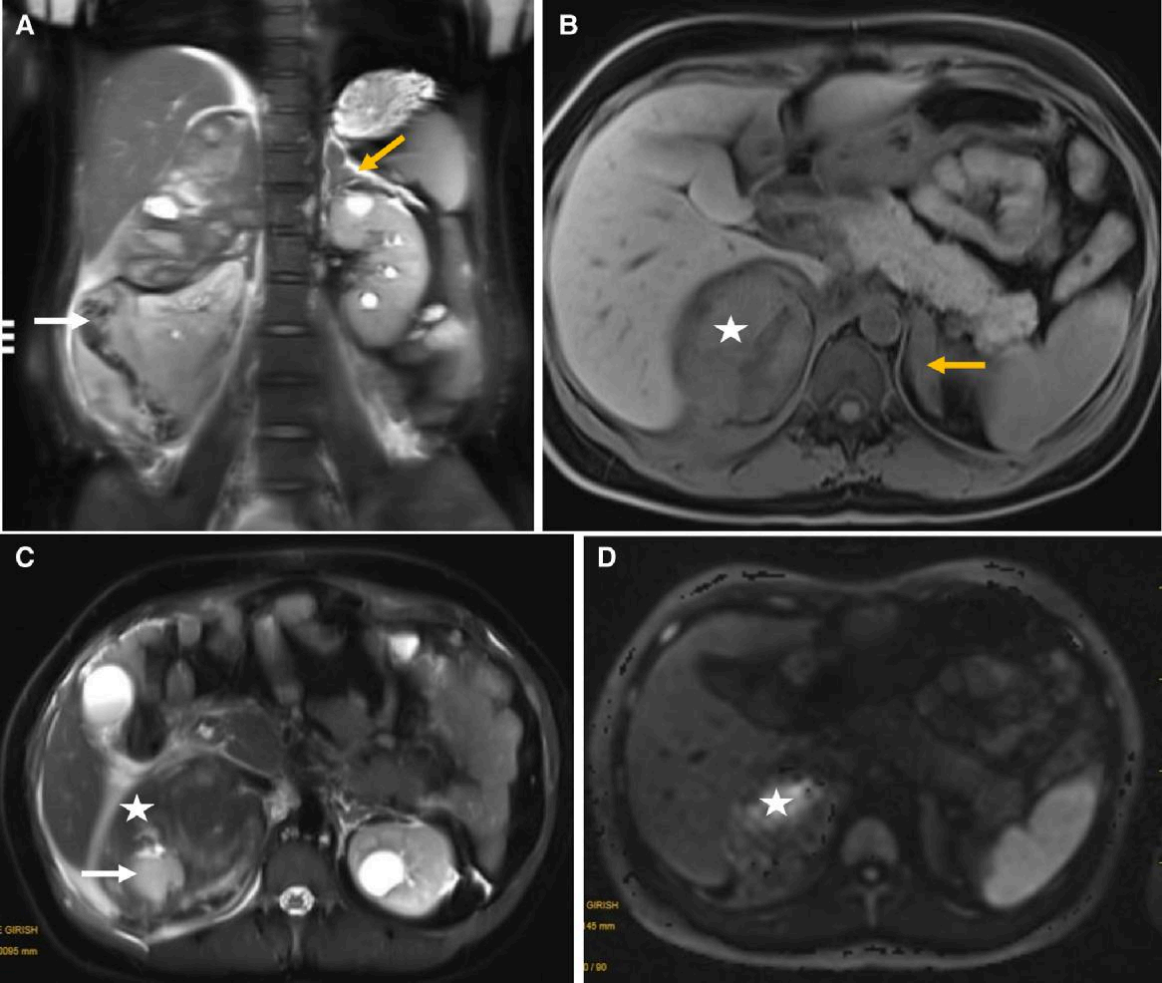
Magnetic resonance imaging showing a well-defined lesion (8.7 × 8.2 × 11 cm) in the right suprarenal area that was heterogeneously hyper-isointense on T2-weighted half Fourier single-shot turbo spin-echo coronal image (A), hyperintense with no fat suppression on axial T1-weighted fat saturation sequences (B) with breach in the posterior capsule with fluid/clots in the right posterior perinephric and pararenal space suggestive of right adrenal hemorrhage (white arrows) (A,C). Corresponding patchy areas of diffusion restriction representing acute hemorrhage (white star; B-D) with adjacent subacute hemorrhage and clots. The left adrenal gland is homogenously iso-hypointense and enlarged (4.4 × 1.2 × 3.1 cm), suggesting left adrenal hyperplasia (yellow arrows).
